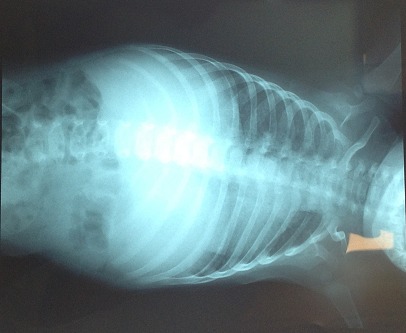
Radiographie thoracique objectivant des micronodules diffuse sur les deux champs pulmonaires évocateurs d'une miliaire tuberculeuse au cours de la première hospitalization
